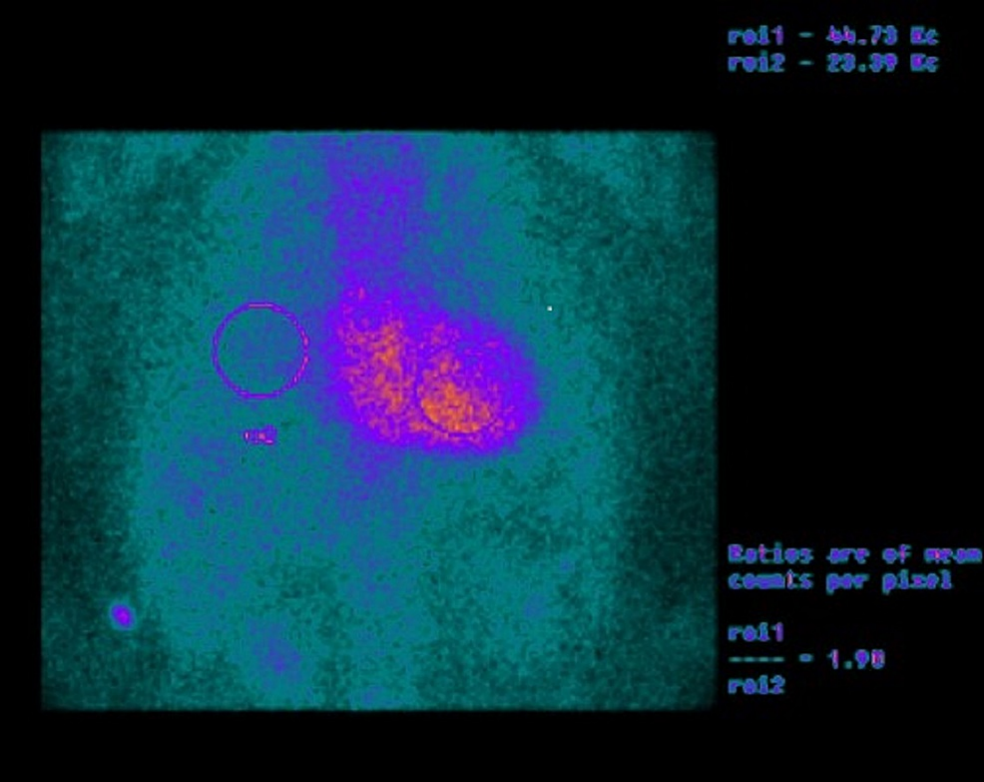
Nuclear imaging of 99mTc-PYP planar scintigraphy showing significant uptake of cardiac 99m Tc-PYP in the myocardium compared to the ribs. Circular target ROI are drawn over the heart on the planar images as well over the contralateral chest. A H/CL ratio is calculated as the fraction of heart ROI mean counts to contralateral chest mean counts. H/CL ratios of >1.5 are classified as ATTR positive at one hour post-injection. 99mTc-PYP: 99mTechnetium pyrophosphate; ATTR: Transthyretin; H/CL ratio: Heart-to-contralateral ratio; ROI: Regions of interest.
